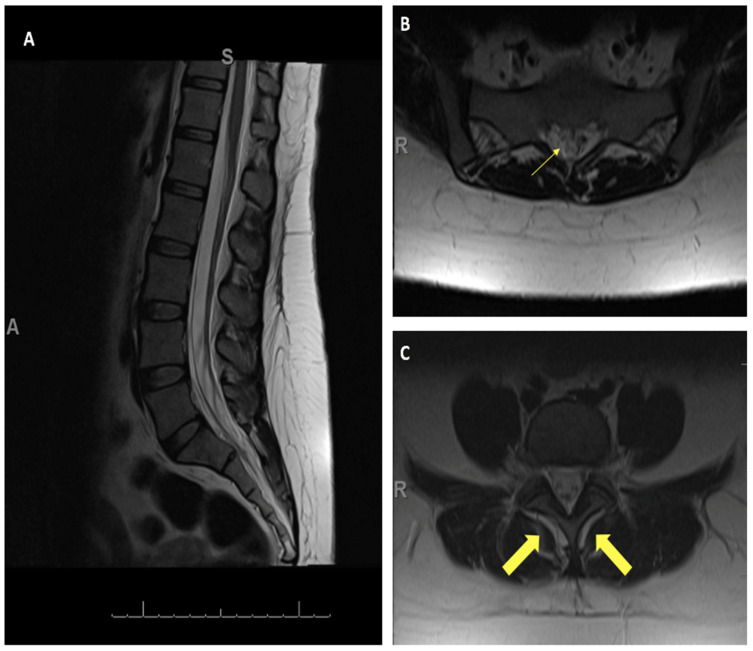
MRI T2 of the lumbar spine. (A) Sagittal image (A: Anterior, S: Superior) and (B,C) axial images showing the area of concern for CSF tracking out of the thecal sac (thin arrow) and CSF signal surrounding the thecal sac and surrounding spinal musculature (thick arrows) (R: Right side).
